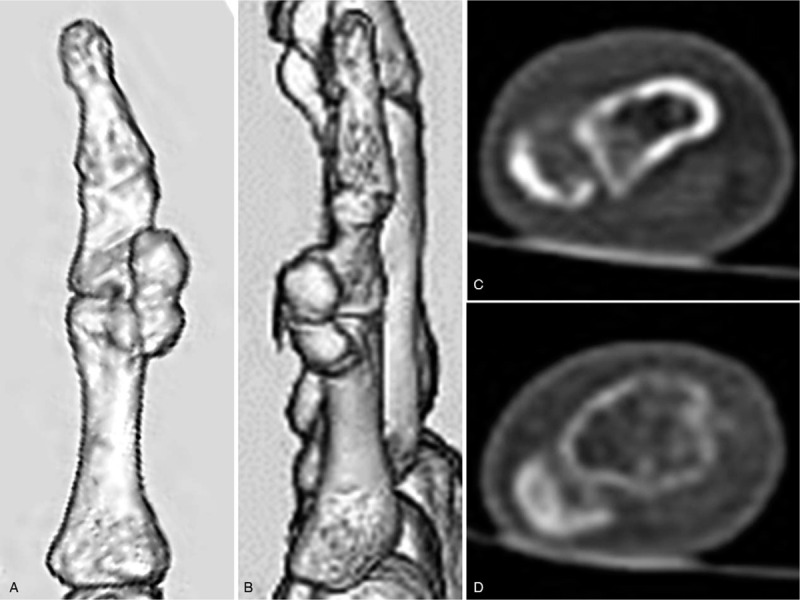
(A) Frontal and (B) lateral 3-dimensional computed tomography views of the left fifth finger, showing subcutaneous bony prominences on the ulnar aspect of the middle and proximal phalanx. Cross-sectional image of the (C) middle and (D) proximal left fifth phalanges, showing a well-defined soft tissue mass overlying the ulnar side of the middle and proximal phalanges with evidence of calcification; the lesion is well separated from the adjacent bone and there is no periosteal reaction or bony destruction.
